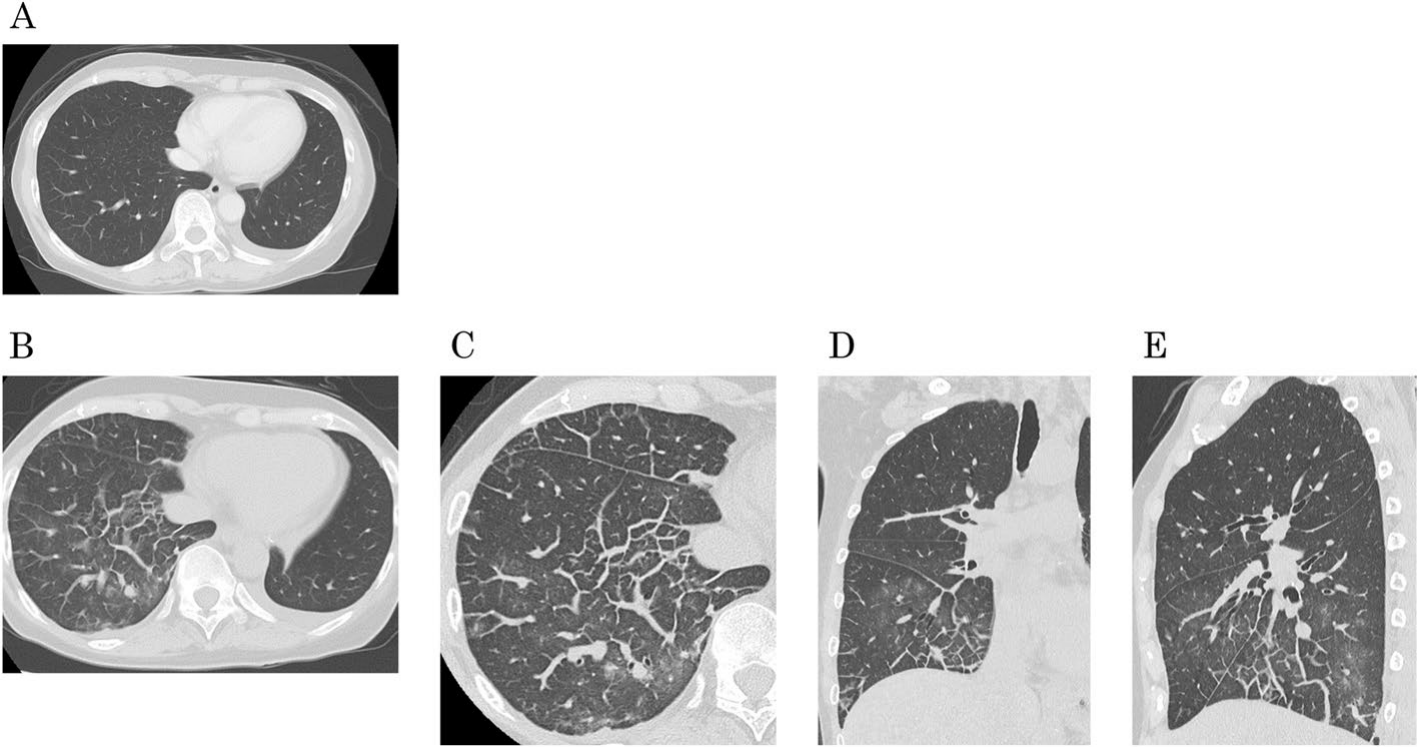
A Computed tomography (CT) of chest showed normal post-operative findings. B Axial computed tomography (CT) showed severe lymphostasis. C Axial, (D) Coronal, and (E) Sagittal view of high-resolution chest CT showed severe lymphostasis
